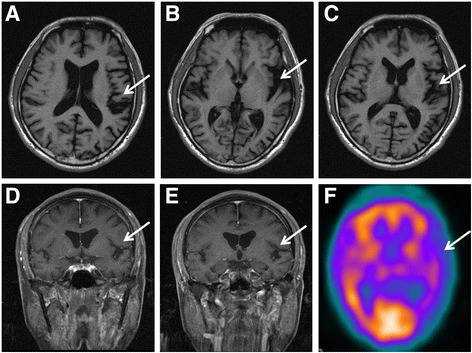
Brain MRI of the index patient. a-e T1WI MRI series showed focal atrophy in the posterior frontal premotor cortex (a-c, arrows) and superior temporal gyrus (d, e, arrows). f SPECT revealed decreased metabolism in left temporal and occipital cortex (arrows)
